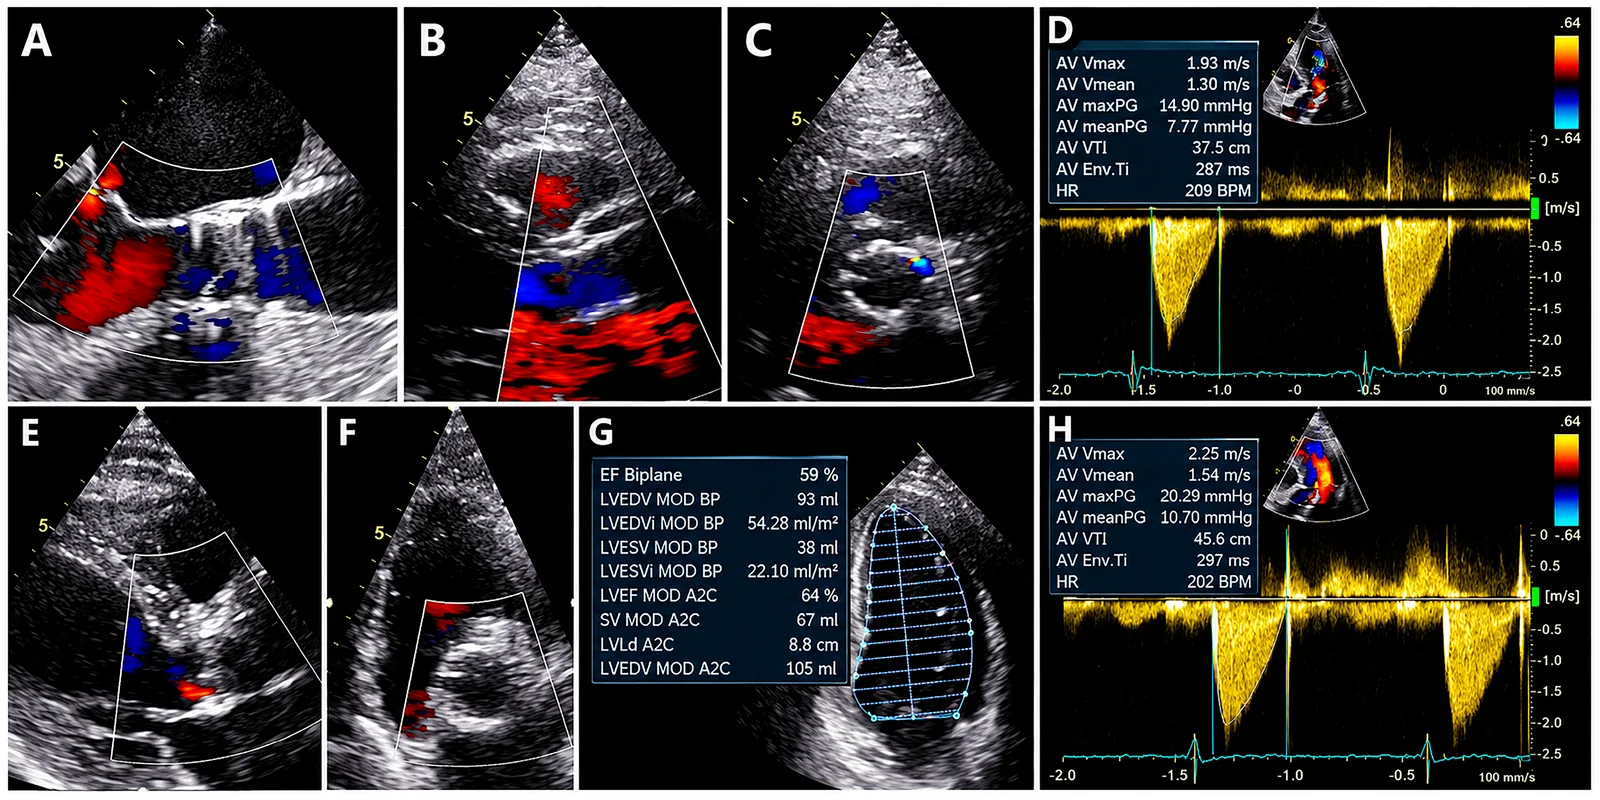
Echocardiographic assessment after TAVI: (A–C). Intraprocedural transesophageal echocardiography following valve implantation, demonstrating appropriate prosthetic valve position and function with trivial paravalvular regurgitation; (D) transthoracic Doppler echocardiography at 1-month follow-up demonstrating preserved prosthetic valve hemodynamics; (E–H) transthoracic echocardiographic assessment at 2-year follow-up, demonstrating preserved left ventricular systolic function and stable transcatheter valve function with low transprosthetic gradients.
